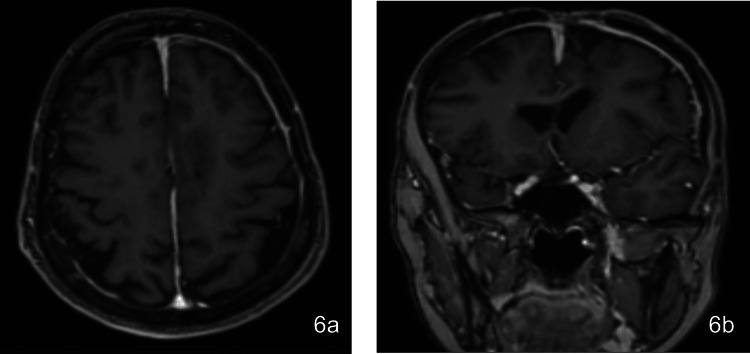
Postoperative MRI at six months in the (a) axial and (b) coronal planes six months posthospital discharge showing resolution of abscess and healing of the closed calvarium
